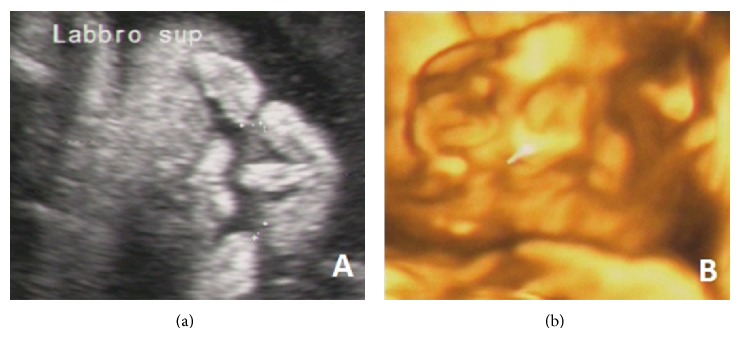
Bilateral cleft lip at 2D (a) and 3D (b) prenatal ultrasonographic scan at 19 and 28 weeks of pregnancy, respectively.
